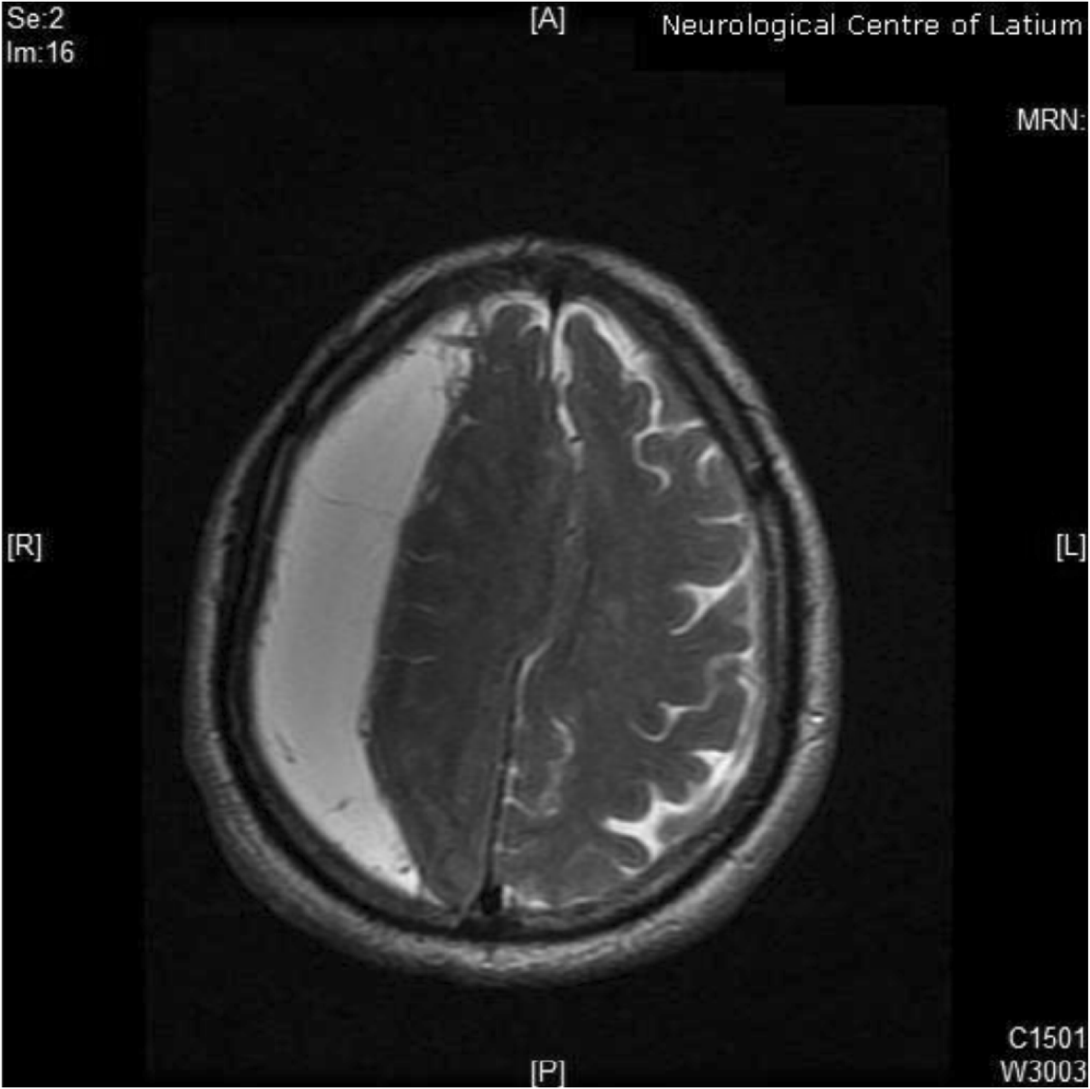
Axial T2-weighted slice of cerebral MRI. A voluminous right frontal, temporal and parietal subdural haematoma with a diameter of 28 mm was present with ipsilateral ventricle compression and an 8 mm shift of median line. A minimal amount of haematic shedding (3 mm diameter) was also present in left frontal subdural space. Some periventricular hyperintense areas were observed as probable expression of microvascular pathology.
